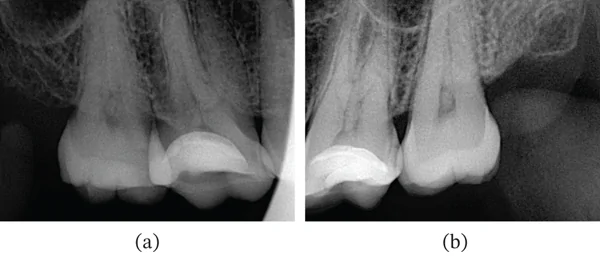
Periapical radiograph showing MIH‐affected molars with overlay restorations on (a) 16 and (b) 26.
